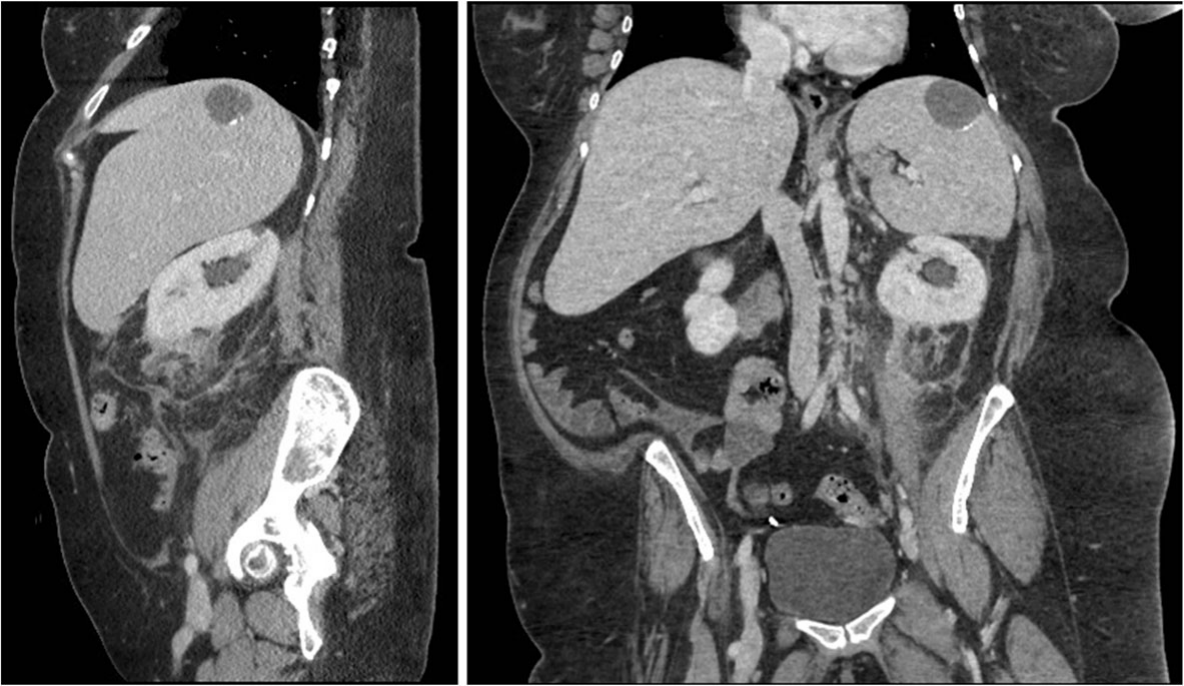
Coronal and sagittal CT scan demonstrating abnormal mass of left kidney, fat stranding, and hepatospenomegaly. There is a 3.5 cm round low attenuated area with peripheral calcifications in the spleen. Likely relates to prior trauma or infection
